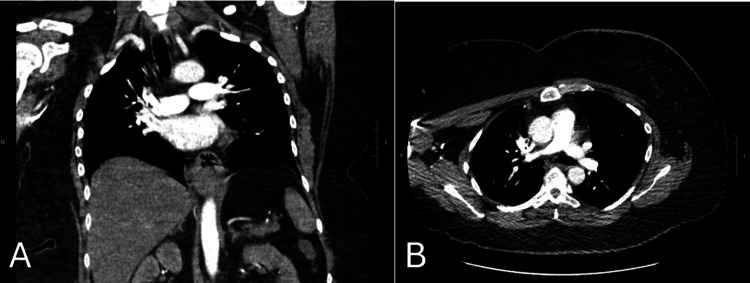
CT pulmonary angiography (A) Coronal and (B) Axial views with contrast opacification of the pulmonary arteries show no intraluminal filling defects.
